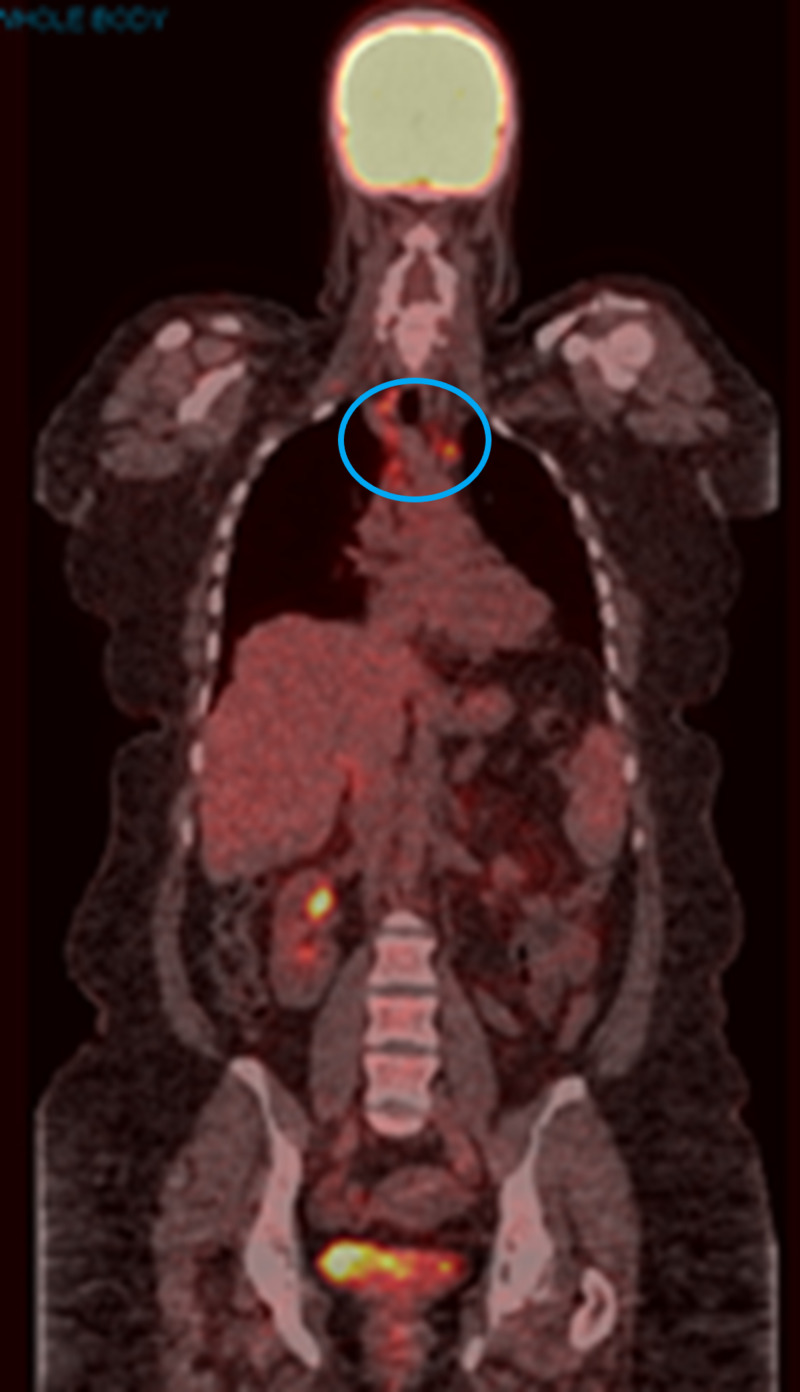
PET/CT scan in November 2018 The image shows persistent mediastinal thoracic adenopathy with increased uptake (blue circle) PET: positron emission tomography; CT: computed tomography
